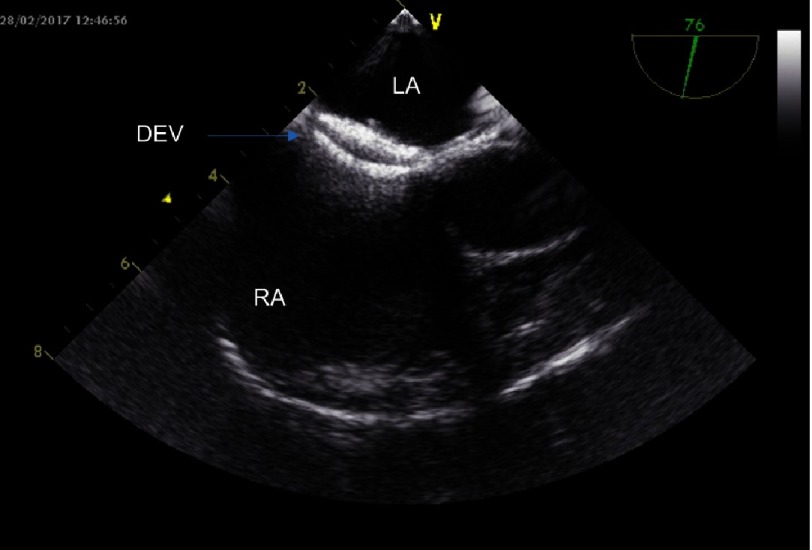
Patent foramen ovale closure after deployment and detachment of the Amplatzer PFO Cribriform Ocluder illustated by transesophageal echocardiography. LA, Left Atrium; RA, Right Atrium; DEV, Amplatzer PFO Occluder device.
